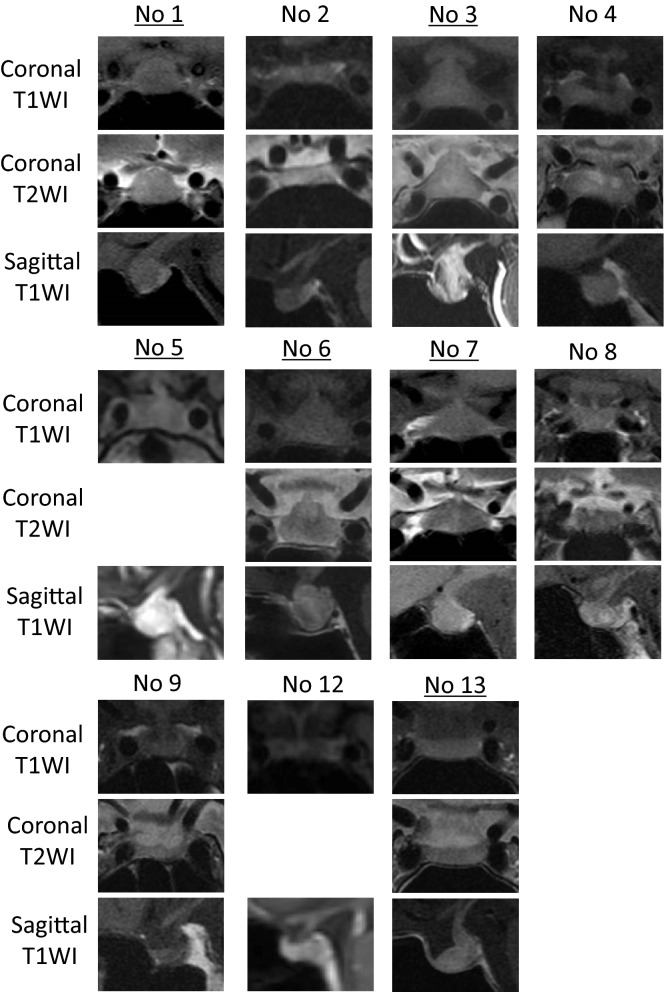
Pituitary MRI of the patients with secondary adrenal insufficiency. MRI analysis revealed enlargement of the pituitary gland in patients No 1, 3, 5–7, and 13 (highlight with underline). Top panels are coronal non-enhanced T1WI, middle panels are coronal T2WI (patents No 5 and 12 lack coronal T2WI), and bottom panels are sagittal enhanced T1 (if not available, non-enhanced T1WI) for each patient.
